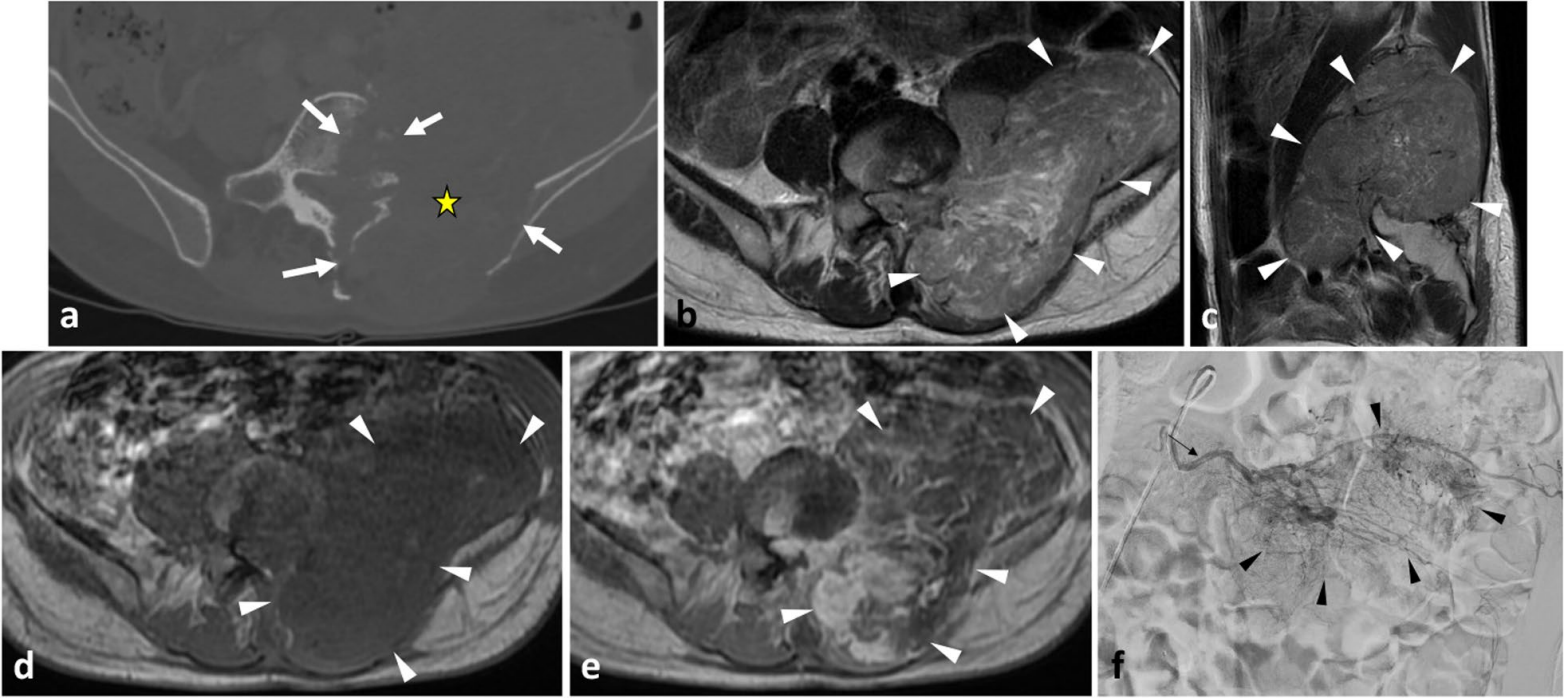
Leiomyosarcoma* of left paraspinal muscles in a 65-year-old male with back pain and genitourinary issues. Contrast-enhanced axial CT scan reconstruction (a) demonstrates aggressive features with moth-eaten lysis of the vertebra and iliac bone (white arrows). The mass extends transversally in both epaxial and in hypaxial muscles (b–e, white arrowheads), and longitudinally along L2 to S1 (c). The mass has heterogeneous high signal intensity on axial (b) and sagittal (c) T2WI, low intensity signal on axial T1WI, and heterogeneous enhancement on contrast-enhanced fat saturation T1WI (white arrowheads) (e). Digital subtraction angiogram (f) of the left 3nd lumbar artery (arrow) shows hypervascularisation of the tumour (white arrowheads). The yellow star shows the epicentre of the mass
